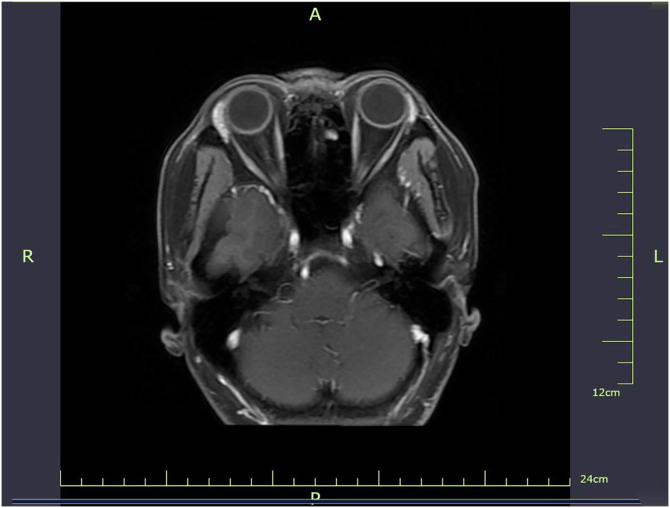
Enhanced MRI (3-weeks after treatment): The frontal soft tissues show slight swelling with heterogeneous slightly prolonged T1 and slightly prolonged T2 signals and ill-defined margins, with moderate enhancement noted on post-contrast imaging. Focal small patchy areas of slightly hyperintense DWI signals are seen in the local soft tissues. The frontal bone demonstrates linear patchy hyperintense DWI signals with insignificant changes in T2 and T1 signals; post-contrast enhancement is not well-defined, with suspected mild enhancement.
